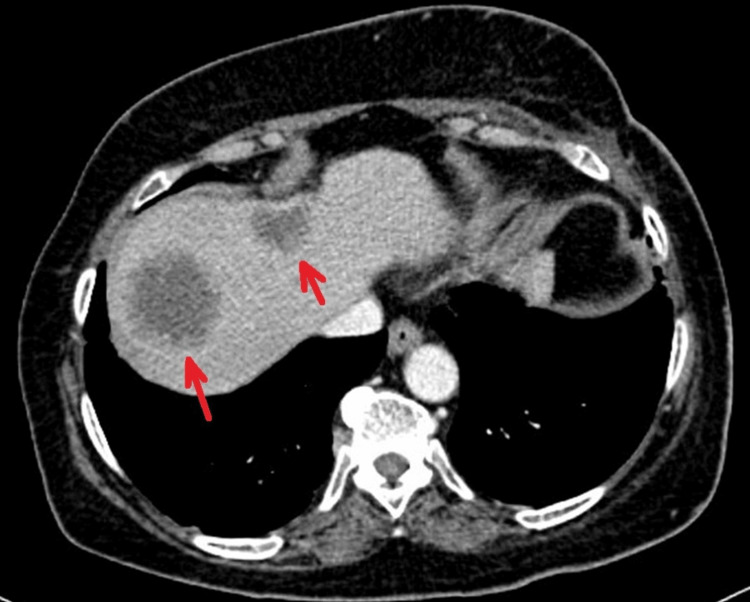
Axial non-contrast CT scan of the upper abdomen demonstrating two new hypodense lesions in the right hepatic lobe (red arrows), consistent with hepatic metastases
